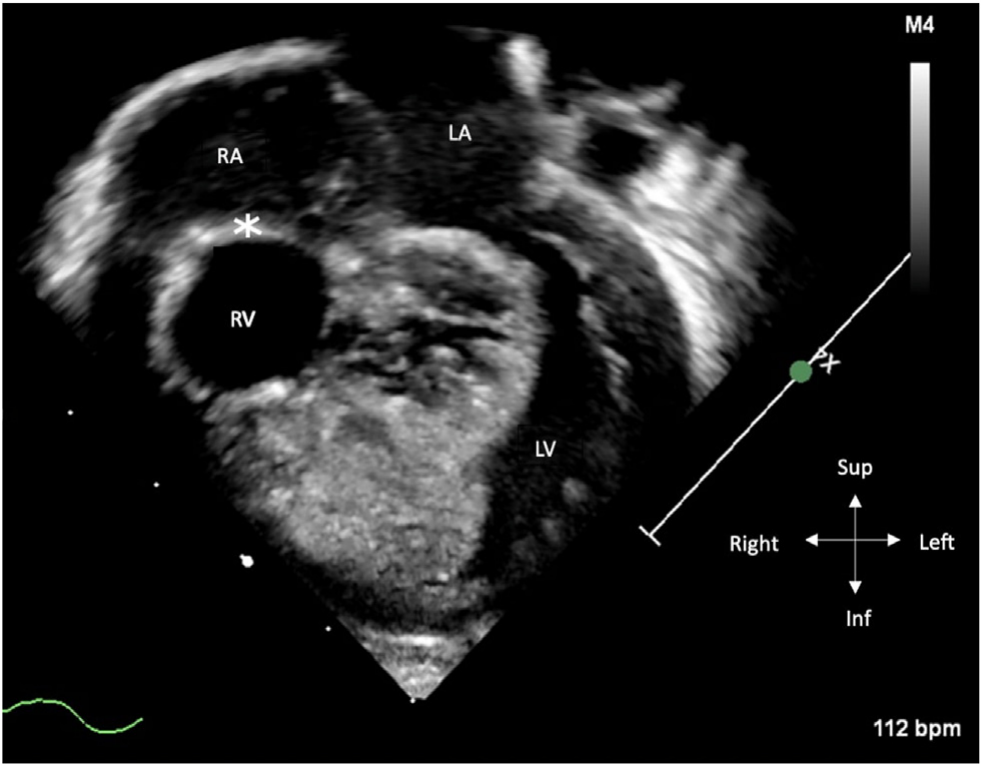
Postnatal two-dimensional transthoracic echocardiogram, apical 4-chamber view, diastolic phase, demonstrates a severely hypertrophied and aneurysmal RV, the ventricular septum encroaching into the LV, and the membranous TA (*asterisk*).
